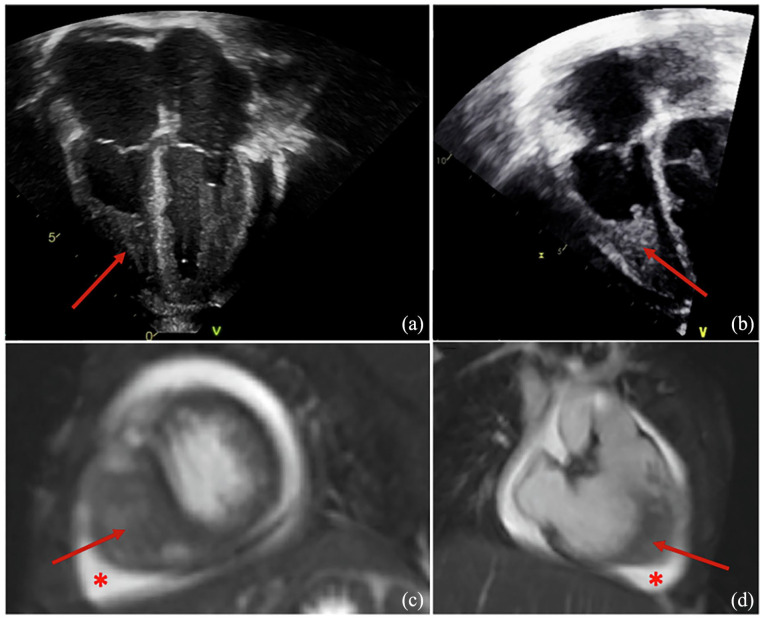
(a and b) Echocardiogram apical images showing RV mass occupying much of the RV apex. (c and d) Cardiac MRI showing RV mass and pericardial effusion. Arrow: RV mass; asterisk: pericardial effusion; RV: right ventricle; MRI: magnetic resonance imaging.
